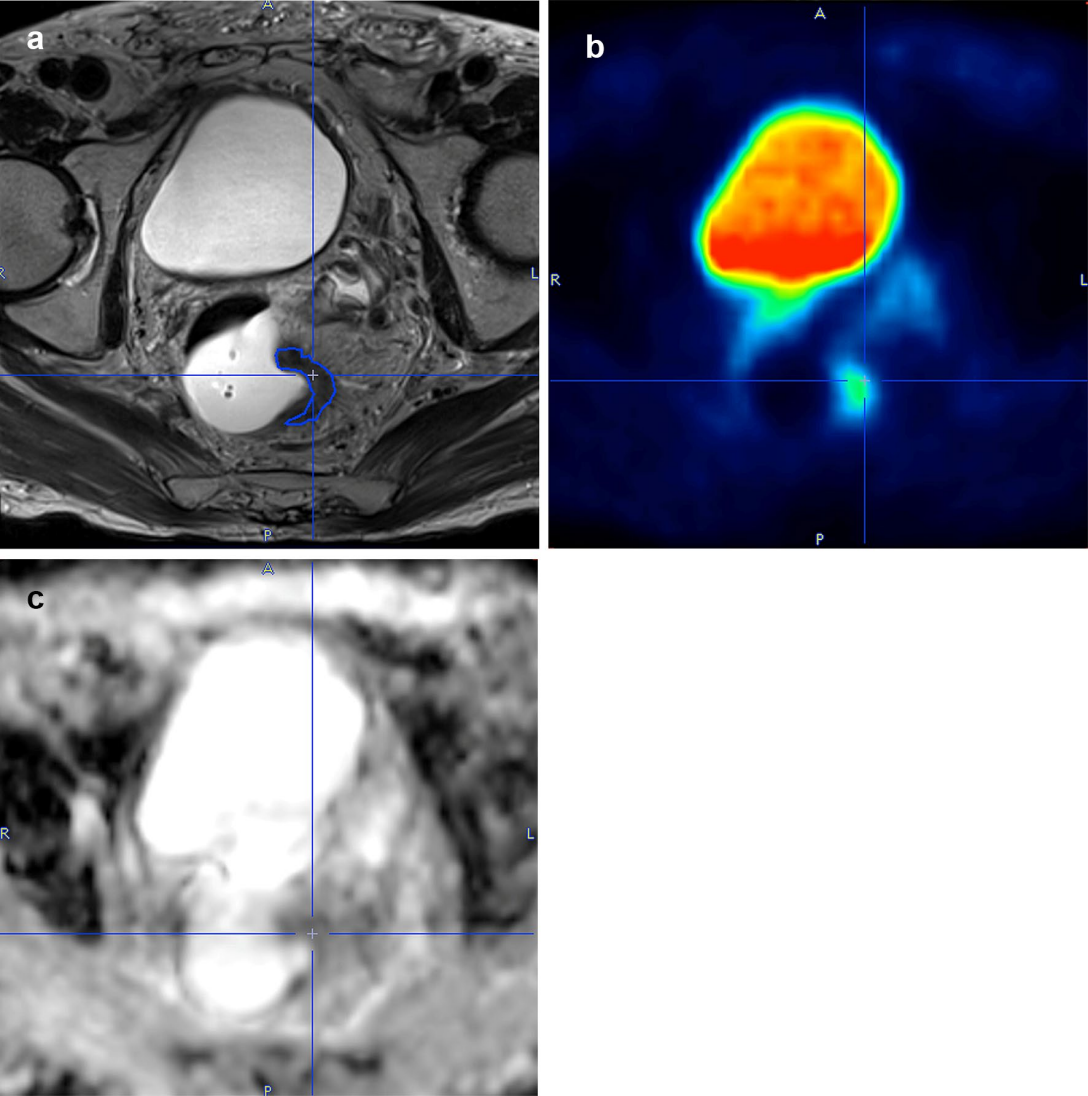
Delineation of the volume of interest with PMOD in a mid-rectal cancer on T2w axial-oblique images (a) and the corresponding PET images (b) and ADC maps (c). *T2w* T2-weighted; *PET* positron emission tomography; *ADC* apparent diffusion coefficient
